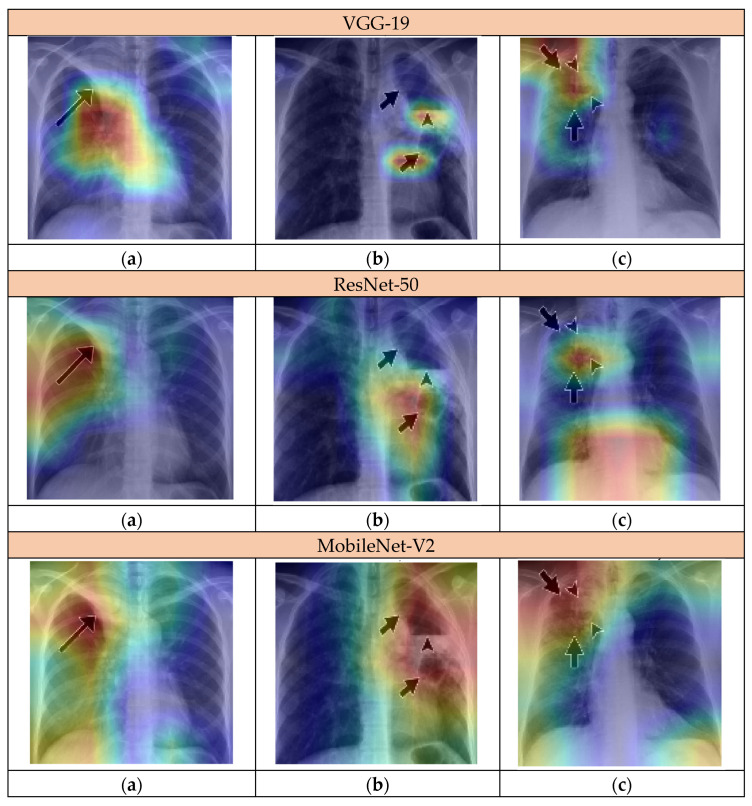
Grad-CAM visualizations on tuberculosis radiographs with expert-annotated radiological findings. (a) A 41-year-old woman with airway involvement due to primary tuberculosis. The arrow indicates a right upper lobe collapse caused by proximal airway obstruction; (b) A patient with two cavitary lesions in the left lung (large arrows) and an air–fluid level inside the larger lower cavity (small arrow); (c) A 50-year-old patient with post-primary tuberculosis, showing characteristic radiological findings.
